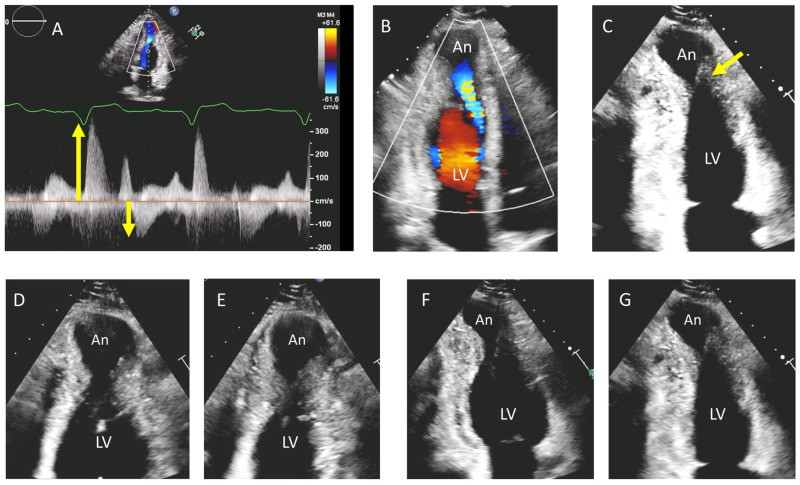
Apical HCM with aneurysm. Modified apical views may be necessary to identify the site of mid-ventricular LV obstruction and confirm the systolic expansion of the apical aneurysm. In this case the Doppler (A) demonstrates an early systolic and early diastolic paradoxical flow (arrows). (B) The colour Doppler facilitates the identification of the mid-ventricular LV obstruction. (C) An adapted two-chamber view shows the obstruction (arrow) and the apical aneurysm (An). Diastolic (D) and systolic (E) frames of the same case (four-chamber view) and diastolic (F) and systolic (G) frames (two-chamber view) demonstrating expansion of the apical cavity in systole.
